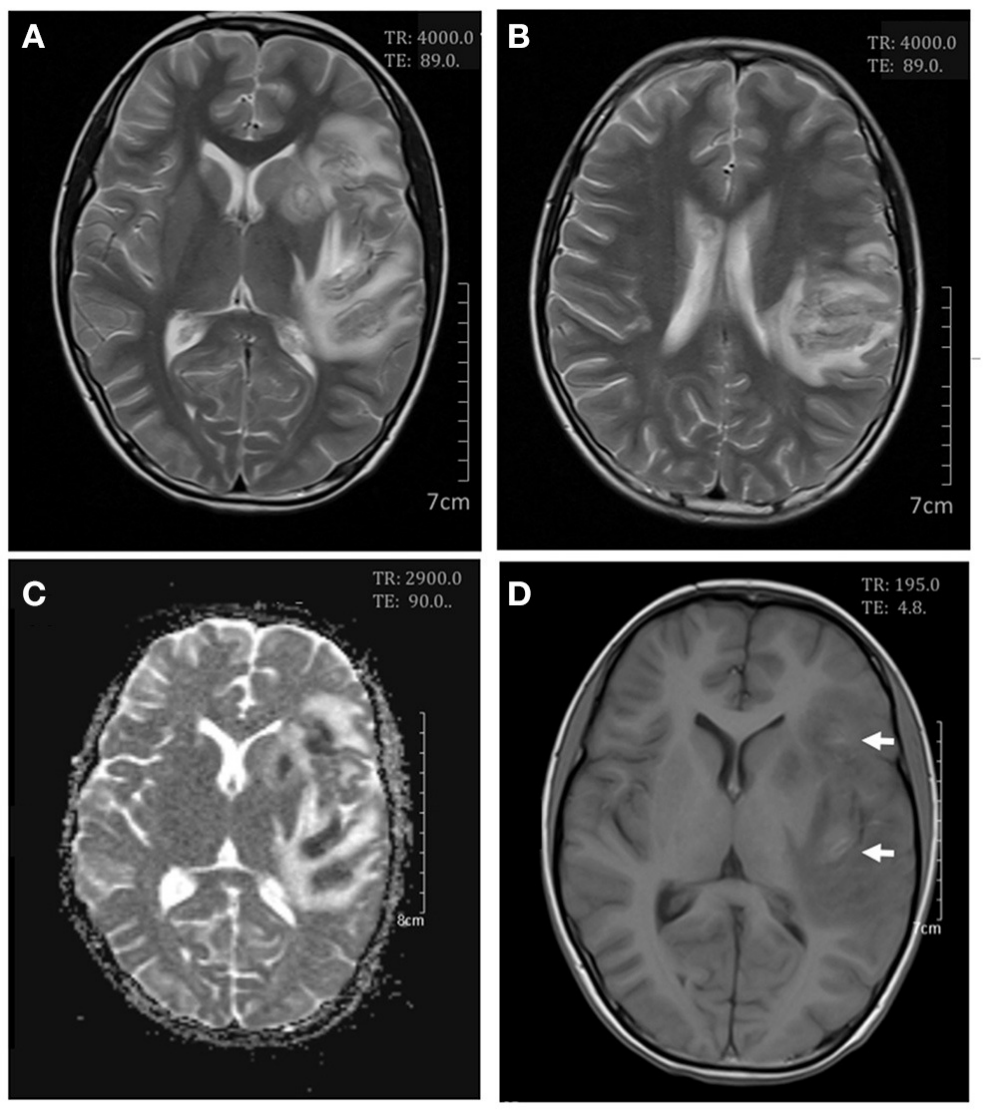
Cerebral magnetic resonance imaging on day 13. Axial T2-weighted scan showed an increase in white matter swelling (A,B). Apparent diffusion coefficient maps demonstrated infarcts in the left lentiform nucleus and the frontal, temporal, insular, and parietal lobes (C). Axial T1-weighted scan showed small areas of hemorrhages in the frontal and insular lobes (D).
